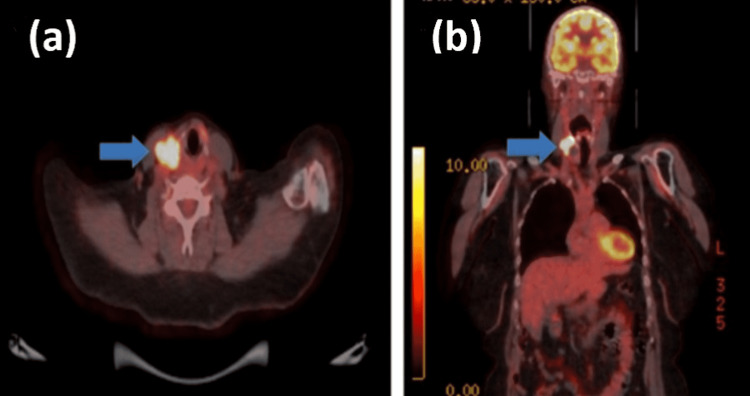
FDG-PET CT Transverse (a) and coronal (b) sections demonstrating a solitary FDG-avid lesion (blue arrow) in the right neck closely related to the right thyroid lobe, which was reported as a likely locally advanced malignancy rather than bowel cancer metastasis.
